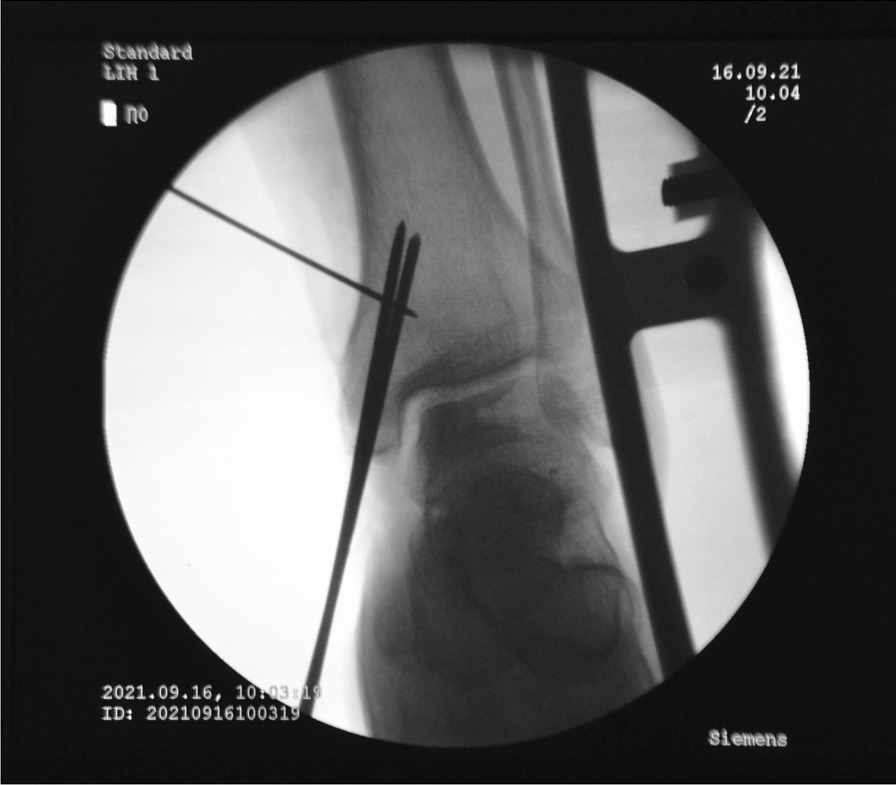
Two 3-mm-diameter Kirschner wires were used to enter the tibia at a 45° angle, and about 2 cm above the tip of the medial malleolusone, one 2.5 mm Kirschner wire was used to enter the tibia and remained vertical to the previous Kirschner wire. X-ray fluoroscopy showed that the position was satisfactory
